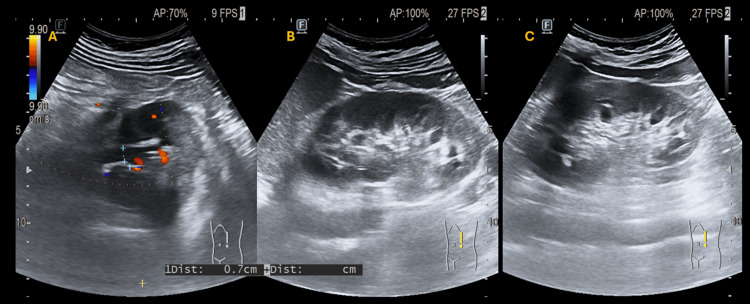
Left kidney seen in one axial image and two longitudinal images. Panel A shows the left renal pelvis on axial view, measuring approximately 7 mm, without calyceal dilatation and with no sonographic evidence of hydronephrosis. Panels B and C show longitudinal ultrasound views of the left kidney, confirming the absence of sonographic hydronephrosis.
